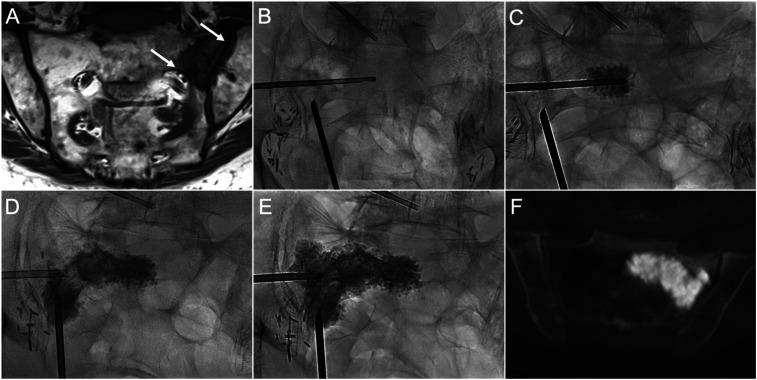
Co-axial sacroplasty of a left sacral ala metastasis. (A) Coronal T1 MRI image shows left ala metastatic lesion with erosions of the sacroiliac joint and S1 foraminal cortical margins (*white arrows*), which increase the risk of cement leakage. (B) PA fluoroscopic image demonstrates transiliac and long-axis guide cannula positioning. A side-opening injection cannula was inserted co-axially through the transiliac guide cannula across the lesion. (C) PA radiograph demonstrates the initial cement deposition. (D) Cement was injected through the long-axis guide cannula after initial sequential transiliac injections were completed. (E) Final distribution of cement after completion of long-axis and an additional transiliac injection in the ala superiorly. (F) Axial CT image shows contiguous cement distribution without evidence of foraminal or sacroiliac joint extravasation.
